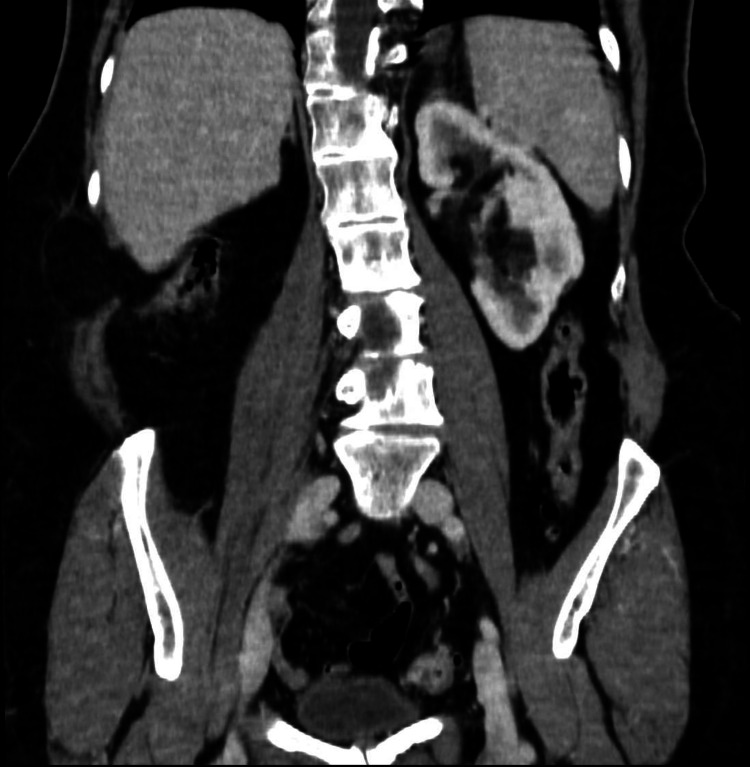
Coronal section of the abdominal CT scan obtained three months after discontinuing antibiotic therapy, showing complete resolution of the lesions seen in Figure 1.
